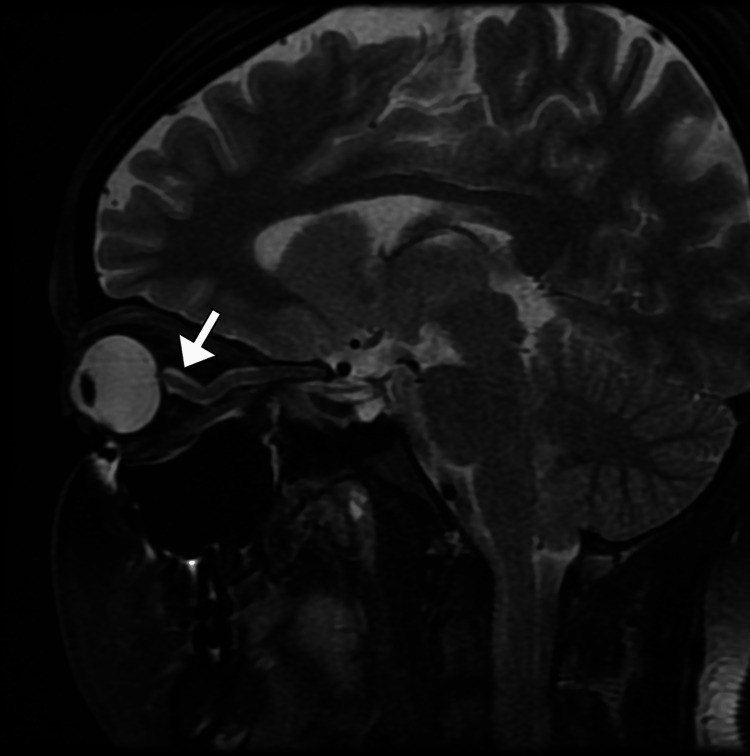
Dilated perioptic subarachnoid space on T2-weighted MRI Sagittal T2-weighted magnetic resonance image (MRI) of the orbit shows dilatation of the perineural (perioptic) subarachnoid space surrounding the optic nerve (arrow). This finding reflects increased cerebrospinal fluid pressure along the optic nerve sheath and is a characteristic imaging feature associated with idiopathic intracranial hypertension.
